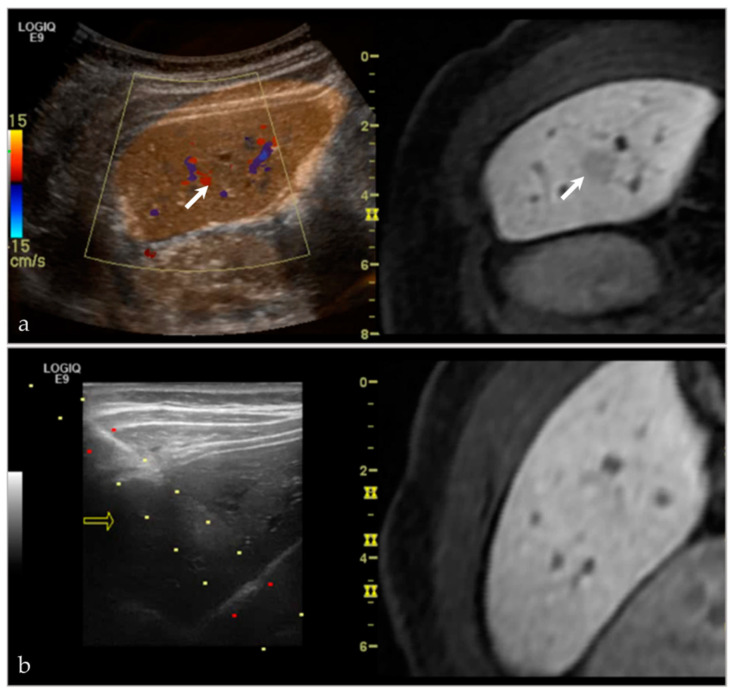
A young female presented with an incidental lesion on MRI. (a) The lesion is difficult to see on MRI alone. However, with ultrasound and the MRI image fused, and imaged side-by-side, it is possible to see a small hypoechoic lesion on ultrasound, corresponding with the hypoenhancing lesion on post-contrast MRI (white arrows). (b) This software was then used to perform an ultrasound-guided biopsy. The lesion subsequently proved to be focal nodular hyperplasia. Please note, the yellow arrow in (b) references in arrow that is used with the needle tracking system during biopsy.
